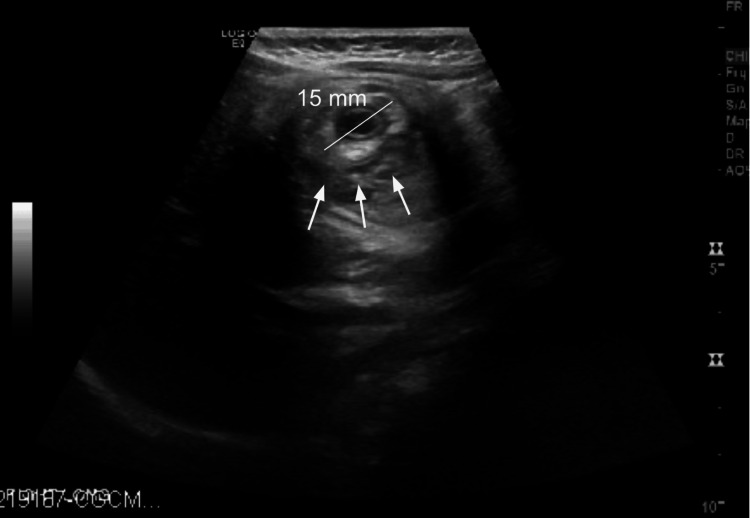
Abdominal ultrasound image on the second presentation The image shows recurrent ileocolic intussusception with a dilated appendix measuring 15 mm in diameter.
